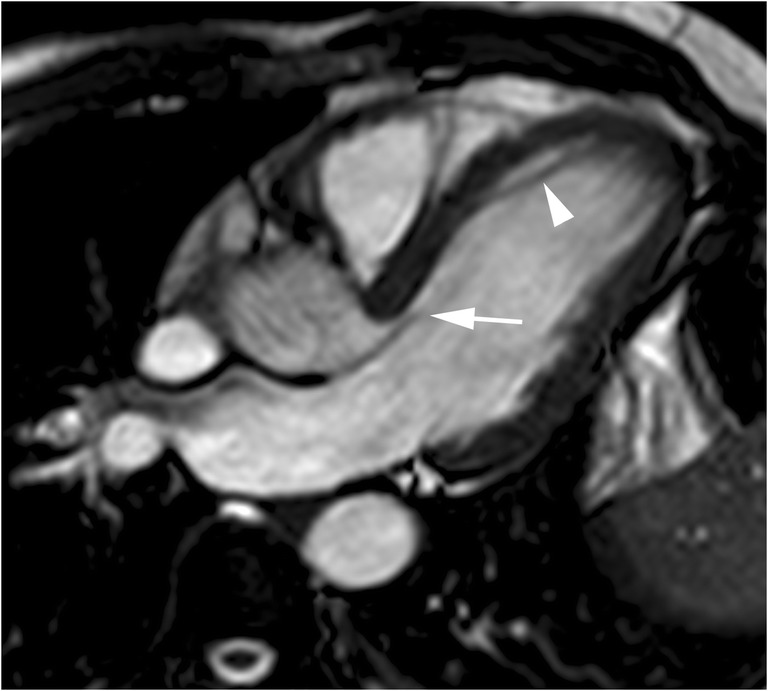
20-year-old man, genotype positive, phenotype negative. End-diastolic horizontal long-axis SSFP cine MR image demonstrates an elongated anterior mitral valve leaflet (arrow) and normal length of posterior valve leaflet. A linear structure (arrowhead) that connects ventricular septum and left ventricular apex, corresponding to aberrant muscular band also can be seen
